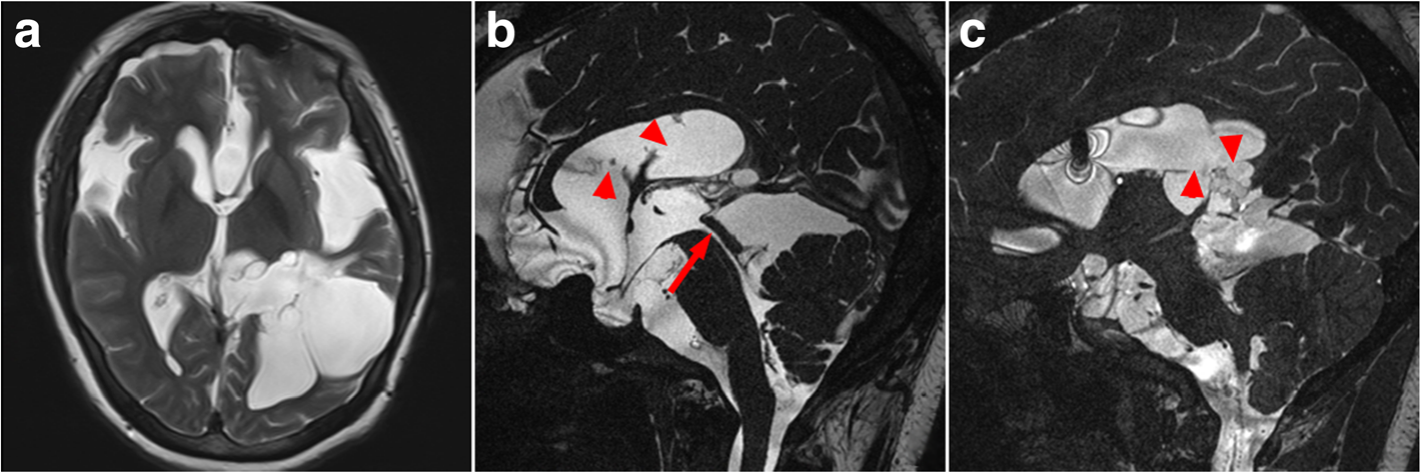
Images obtained a week after the operation. a T2-weighted axial MR image. b and c Cine phase-contrast MR image. b Arrow indicates that satisfactory CSF flow through aqueduct of Sylvius is detected. ▲Indicates the fenestrations of the apical membrane (f_1_ and f_2_). c ▲Indicates two fenestrations of the basal cyst membrane behind the midline (f_3_ and f_4_)
